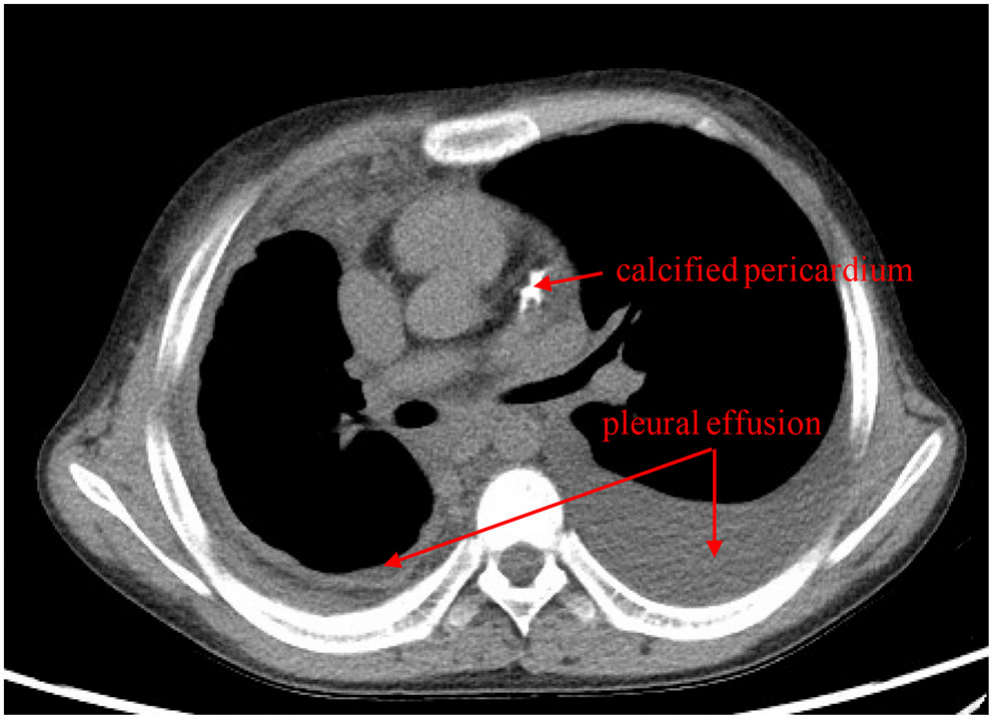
Cardiac computed tomography shows bilateral pleural effusion and a calcified pericardium (red arrows).
